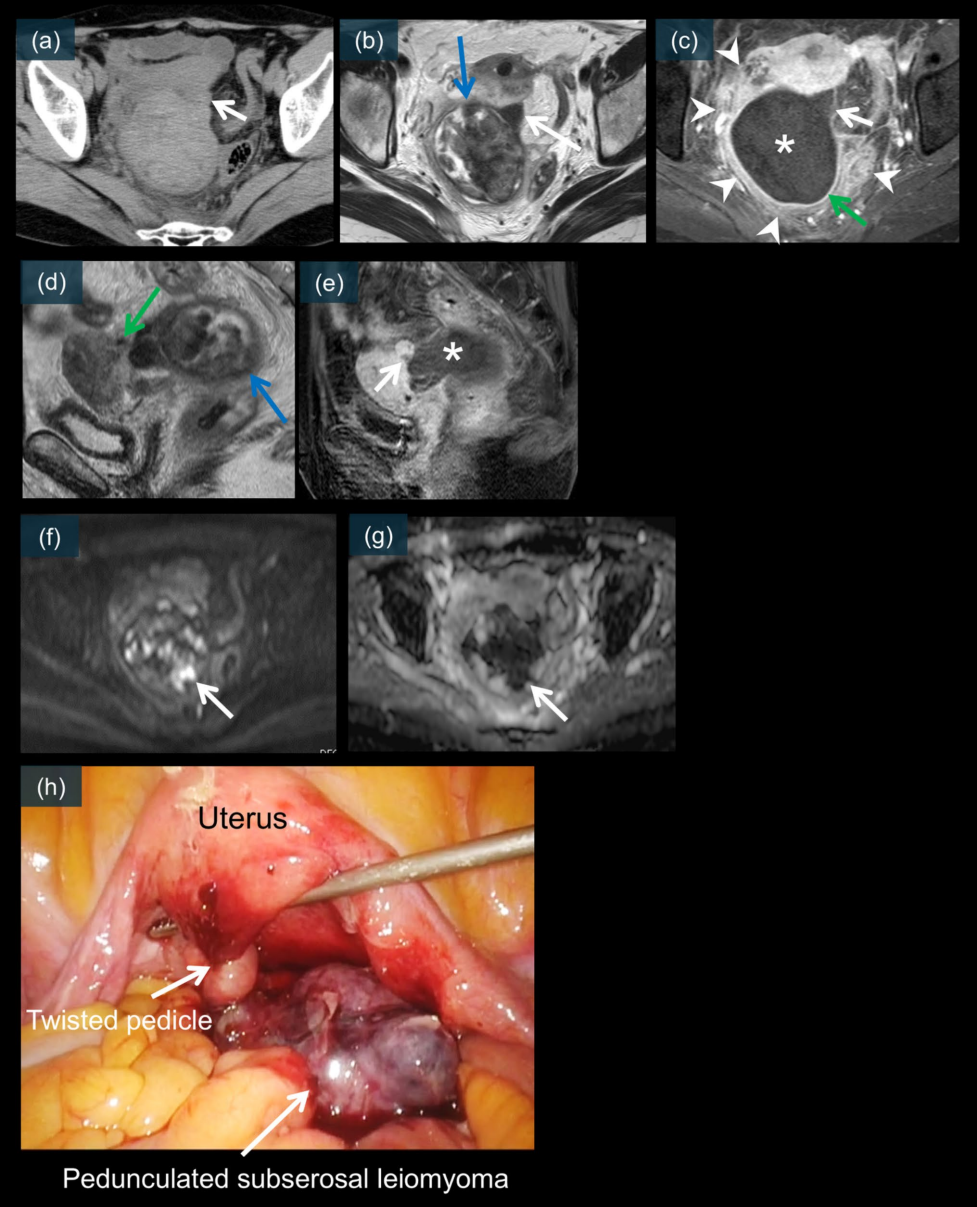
A 66-year-old female. An unenhanced CT scan (a) shows a mass with a long diameter of 7.4 cm in the pelvic cavity, which appears to be partially continuous with the uterus (white arrow). On T2WI (b and d), the structure continuous with the uterus is clearly depicted and can be recognized as an enlarged stalk in the axial view (white arrow). In the sagittal view, a bridging vessel sign is observed (green arrow). The mass shows a heterogeneous internal signal intensity (blue arrows). On the contrast-enhanced images (c and e), neither the stalk nor the mass showed enhancement (white arrows and asterisks). A thin enhanced rim is observed at the periphery (green arrow). The nonenhanced area corresponds to necrosis, and the usual rim of enhancement corresponds to obstructed peripheral veins with edema. There is also enhancement around this area, suggesting inflammation (white arrowheads). DWI/ADC (f and g) show partial diffusion restriction within the mass, corresponding to ischemia and hemorrhagic necrosis (white arrows). (h) is a laparoscopic image showing a subserosal leiomyoma that has undergone a 720° torsion
